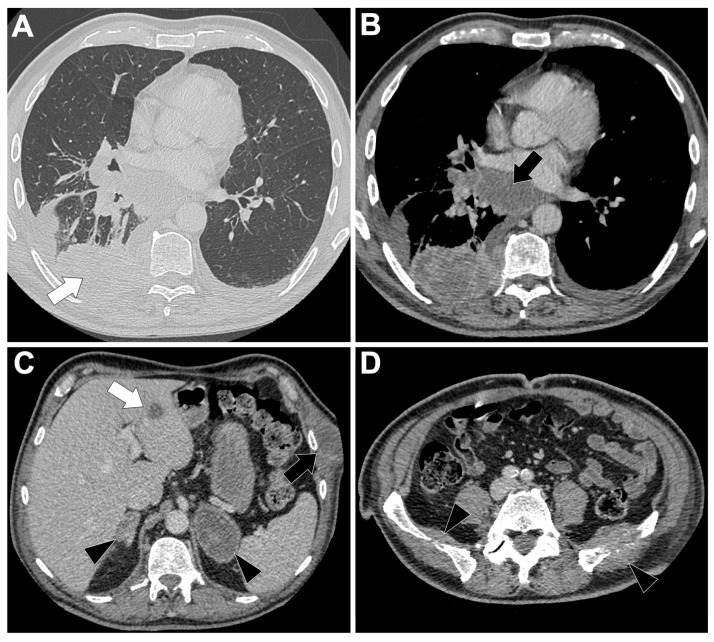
Representative baseline CT imaging findings in patients with KRAS-altered lung adenocarcinoma (LUAD). A 67-year-old man with a heavy smoking history (50 pack-years) presented with a peripheral necrotic mass in the right lower lobe (white arrow) (A), associated with bulky necrotic subcarinal lymphadenopathy (black arrow) (B). Abdominal CT images (C) demonstrated bilateral necrotic adrenal metastases (black arrowheads), a hepatic metastasis (white arrow), and intercostal muscle metastases (black arrow). (D) The patient also exhibited bilateral lytic metastases of the iliac bones (black arrowhead). Molecular analysis identified a KRAS G12C mutation.
